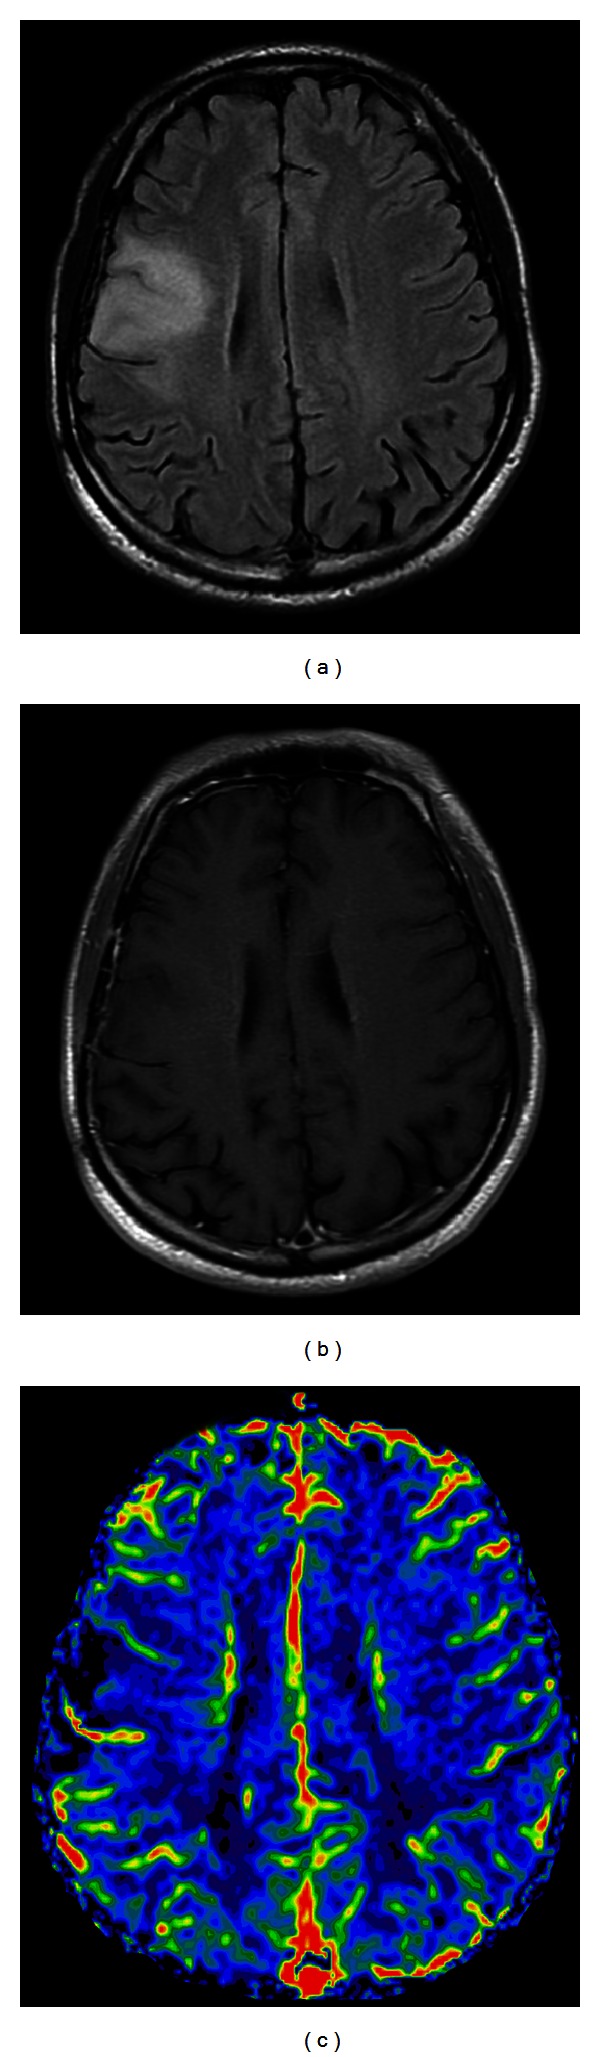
(a) FLAIR image shows a hyperintense low-grade glioma, WHO grade II, without significant contrast enhancement on T1-weighted image (b) or increase of the rCBV (c).
